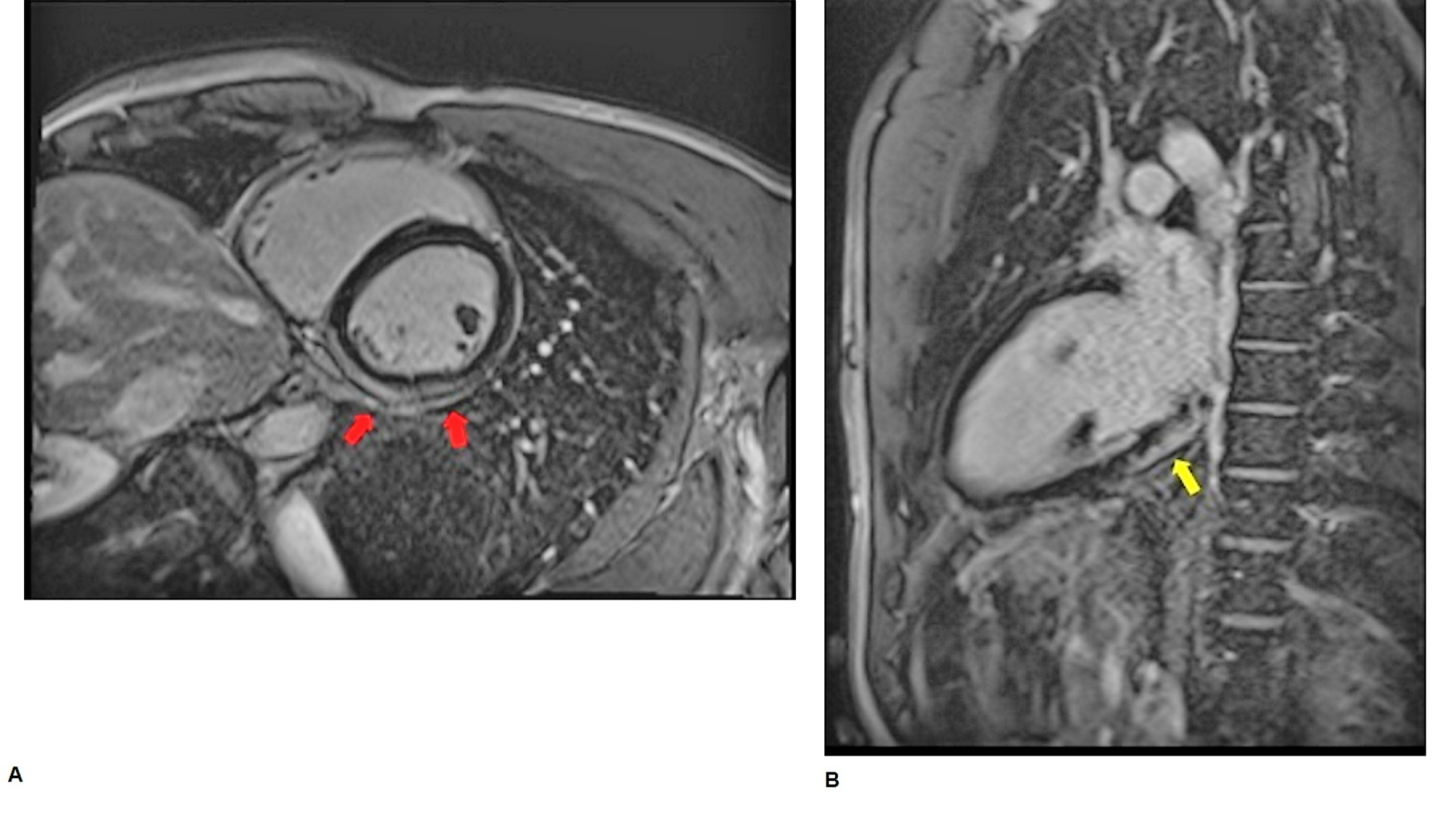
Cardiac magnetic resonance imaging (CMR). A: CMR, axial plane, showing sub-epicardial to mid-myocardial delayed hyper-enhancement involving the basal to mid-inferior and infero-lateral walls consistent with myocarditis (red arrows). B: CMR, coronal plane, showing sub-epicardial to mid-myocardial delayed gadolinium hyper-enhancement involving the basal to mid-inferior and infero-lateral walls consistent with myocarditis (yellow arrow).
